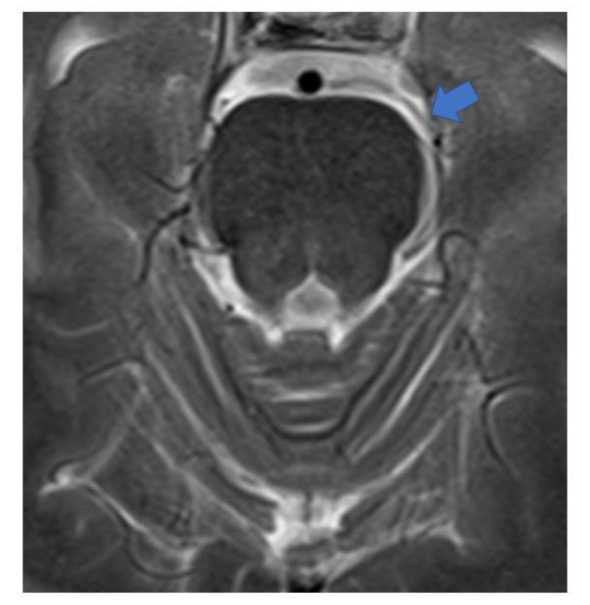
High resolution axial MRI image. The vascular loop runs alongside the left lateral aspects of cranial nerve V (trigeminal) root exit.
